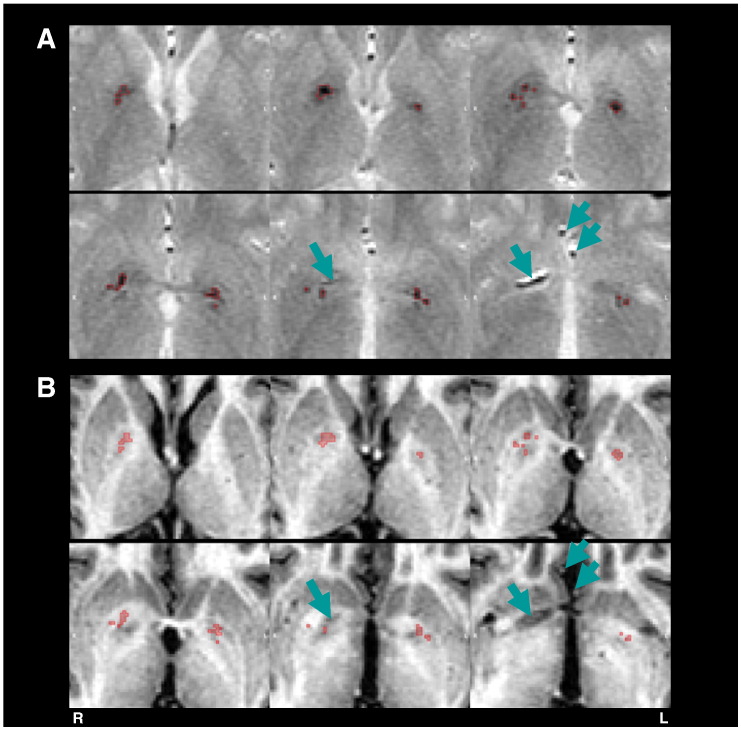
Focal T2*w hypointensities in the basal ganglia of a typical subject. The perimeters of the T2*w hypointensity masks from the rater are indicated by red lines on top of (A) T2*w and (B) co-registered T1w axial slices. This particular subject just exhibits multifocal T2*w hypointensities in the globus pallidus. In more severe cases, T2*w hypointensities are also found in the internal capsule, putamen and caudate. Arrows indicate arterial structures, which mimic T2*w hypointensities on T2*w MRI. However, these features have a branch like appearance and are generally hypointense on T1w MRI.
